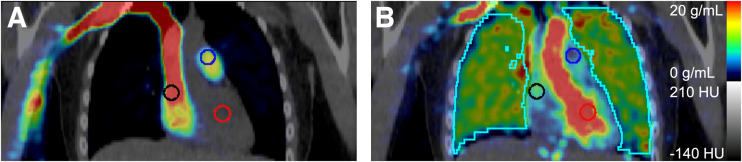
Representative coronal PET/CT images after injecting 207 MBq of ^18^F-NOS, with lung volume of interest (cyan) and 2-cm-diameter spheric blood pool volumes of interest in right atrium (black), pulmonary artery (blue), and left ventricle (red). PET summed uptake is shown from 0 to 15 s after injection (A) and from 37 to 42 s after injection (B). HU = Hounsfield units.
